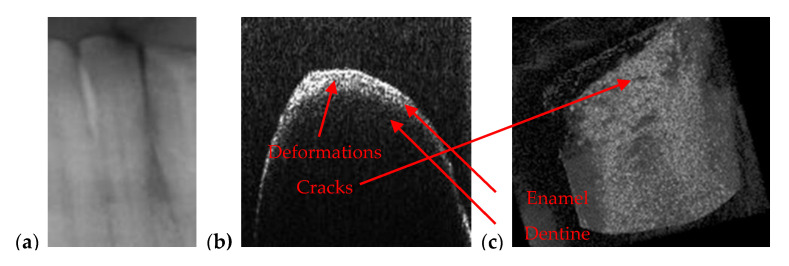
Enamel deformations of an incisive tooth from mandible that cannot be observed on a section cropped from a panoramic radiography (a) but can be clearly remarked both on (b) OCT B-scans and on (c) a 3D OCT reconstruction (performed on the tooth extracted for medical purposes after performing the radiography).
